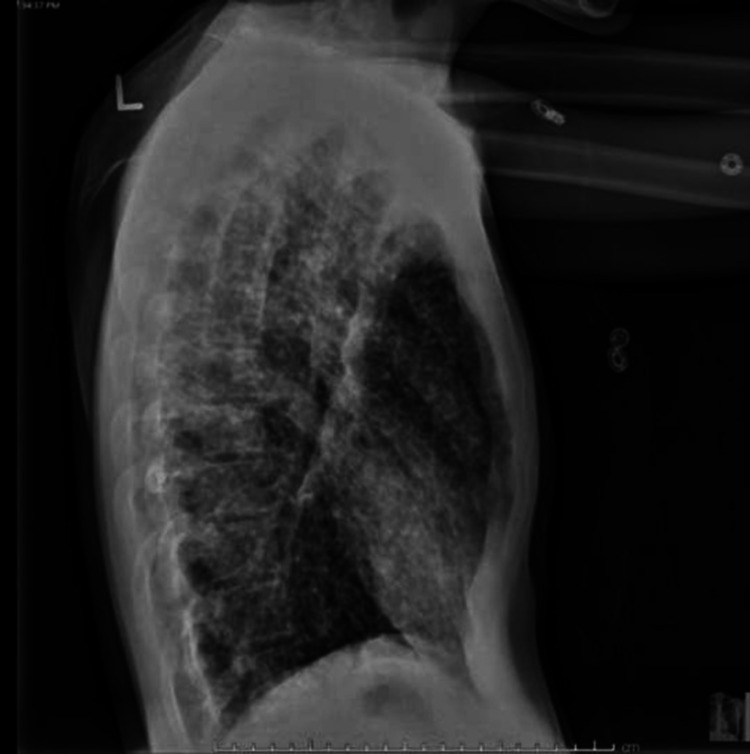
Lateral chest x-ray on admission
